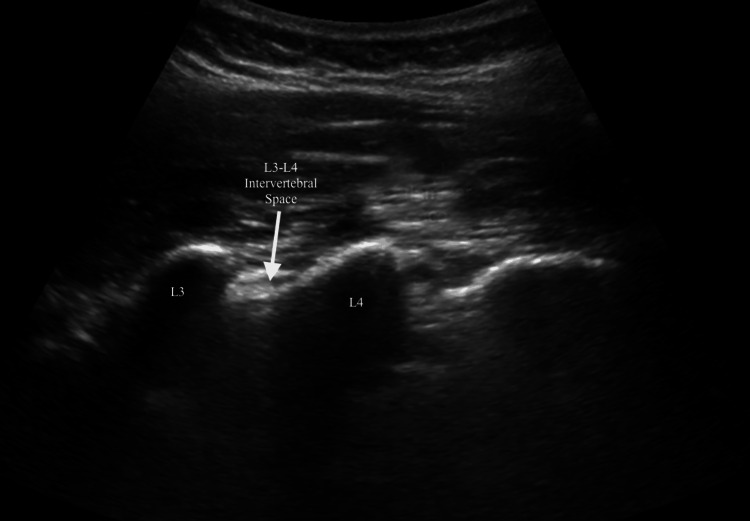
Ultrasound image showing L3-L4 intervertebral space
